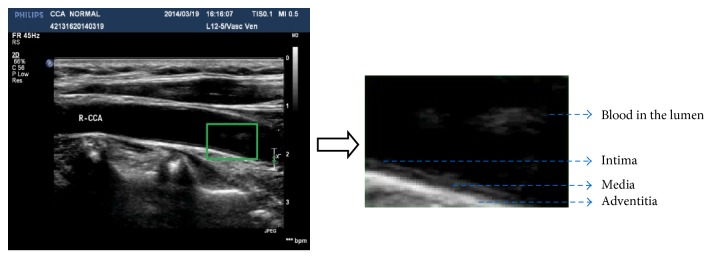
The ultrasound B-mode image scanned from a normal CCA (left) and the magnified region indicated by a green box for different tissue segmentation.
